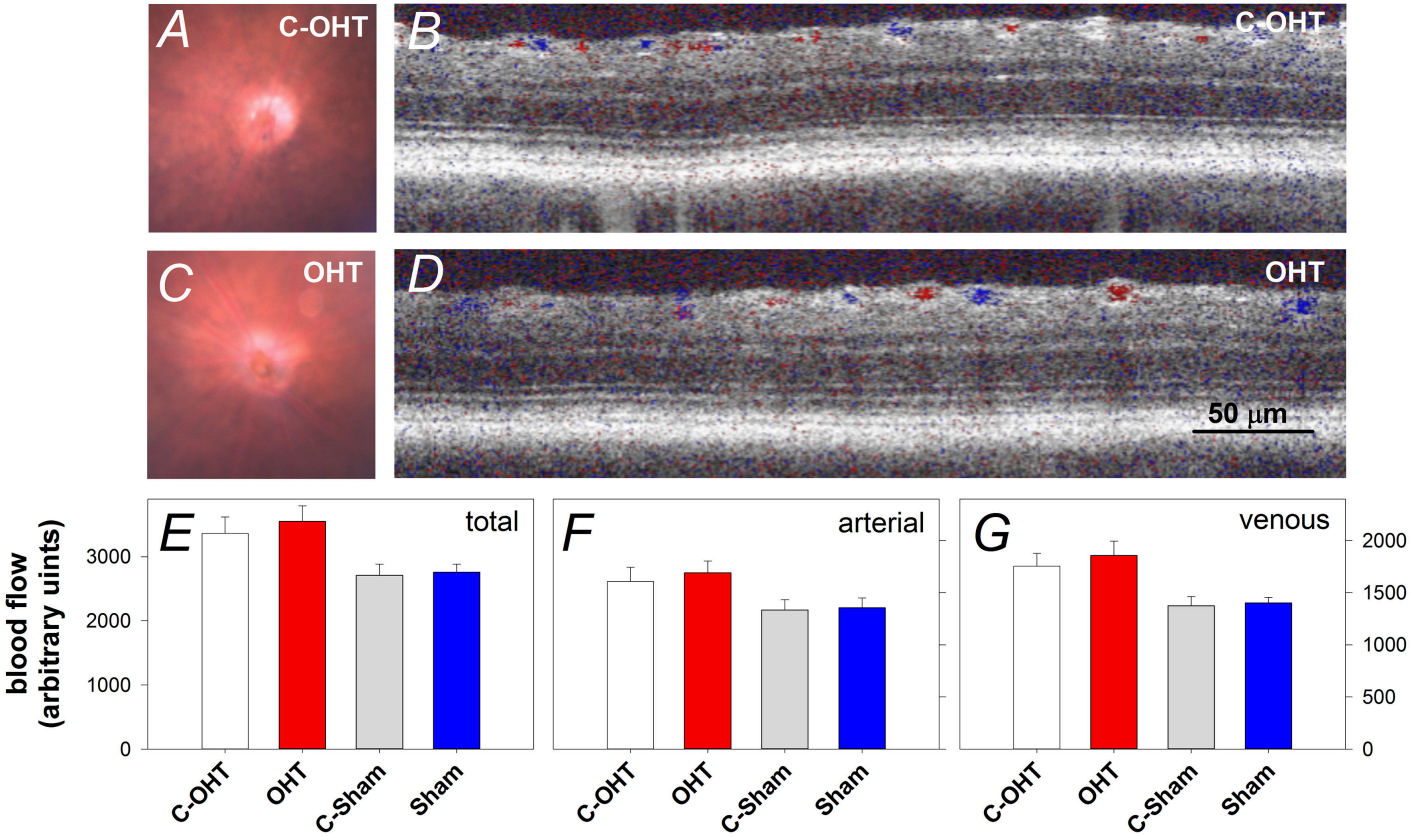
Effect of chronic circumlimbal suture on retinal blood flow. (A,C) Fundus images of a control and the treated eye in the OHT group. (B,D) Representative images of Doppler blood flow annular scan in the same C-OHT and OHT eyes. (E) Group average (± SEM) total blood flow signal in OHT (*n* = 23, red) and Sham (*n* = 17, blue) treated eyes and their contralateral controls (C-OHT unfilled, C-Sham gray). (F) Group average arterial signal. (G) Group average venous signal.
